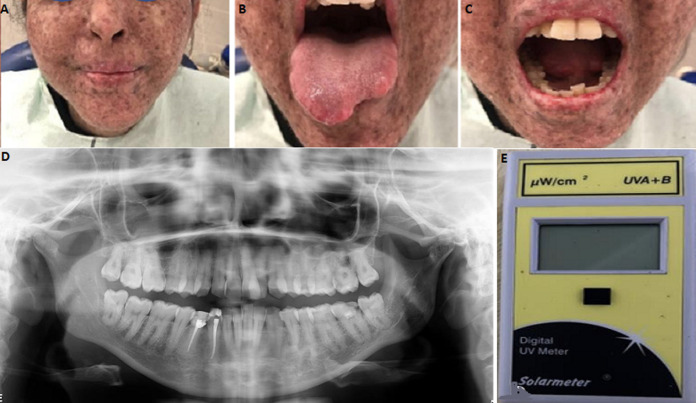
clinical examination of the XP patient: A) multiple hyper pigmented freckles and macules on the face; B) scar due to the resection of a tumour on the tongue; C) limited mouth opening; D) panoramic radiograph showing the number, shape, and position of the teeth; E) patient´s UV meter used to monitor the UV exposure
